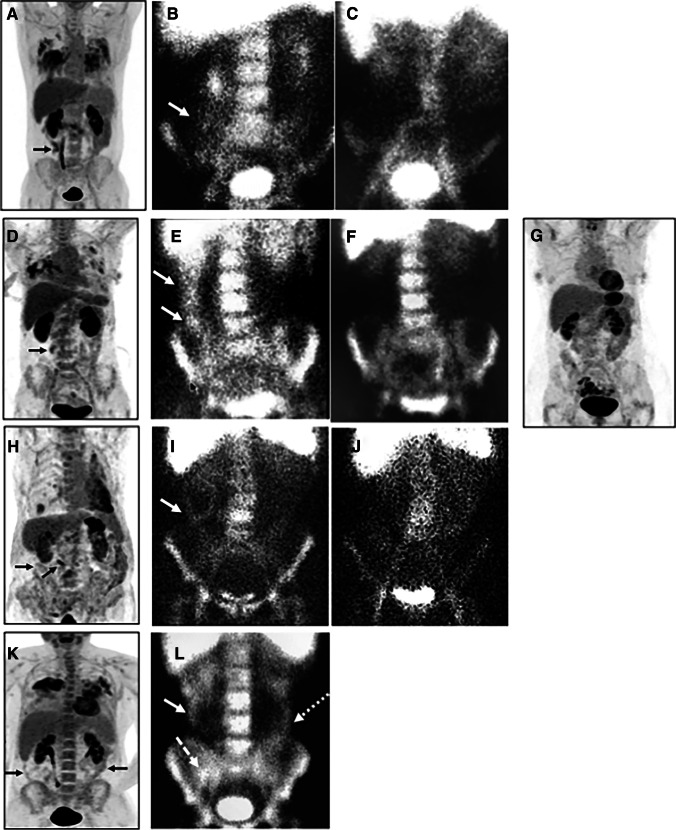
Anterior view of the abdomen was acquired 2 h after intravenous injection of radiolabelled leukocytes in 4 patients at the time of diagnosis of COVID-19 (B, E, I, L) and at recovery (C, F, J). All patients show the presence of radiolabelled autologous leukocytes in the right colon (solid arrows) and in the caecum (dashed arrow) and left colon (dotted arrow) in one case (L). Scans performed after recovery from COVID-19 (C, F, J) do not show any activity in the bowel. Bone marrow activity is also lower at recovery as compared to diagnosis. Maximum intensity projection (MIP) images after [^18^F]FDG of the same patients are also shown at the time of diagnosis (A, D, H, K) and after recovery (G). Activity in the lungs is clearly visible, as well as activity in the bowel (solid black arrows)
